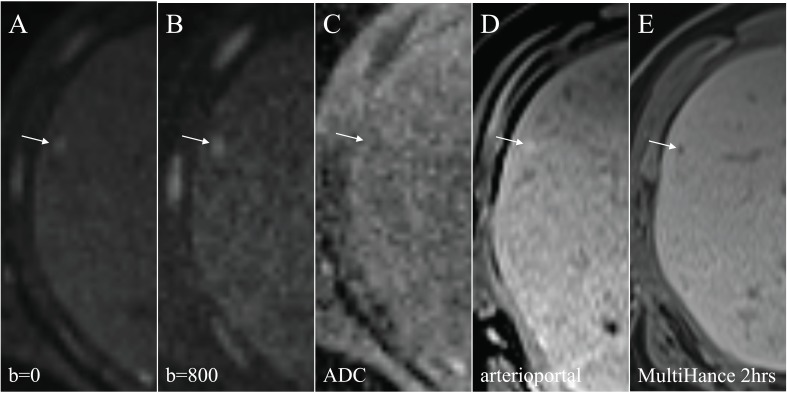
A carcinoid liver metastasis (arrows) is hyperintense and readily detected on DWI, and the intensity is less pronounced on b=0 (A)
